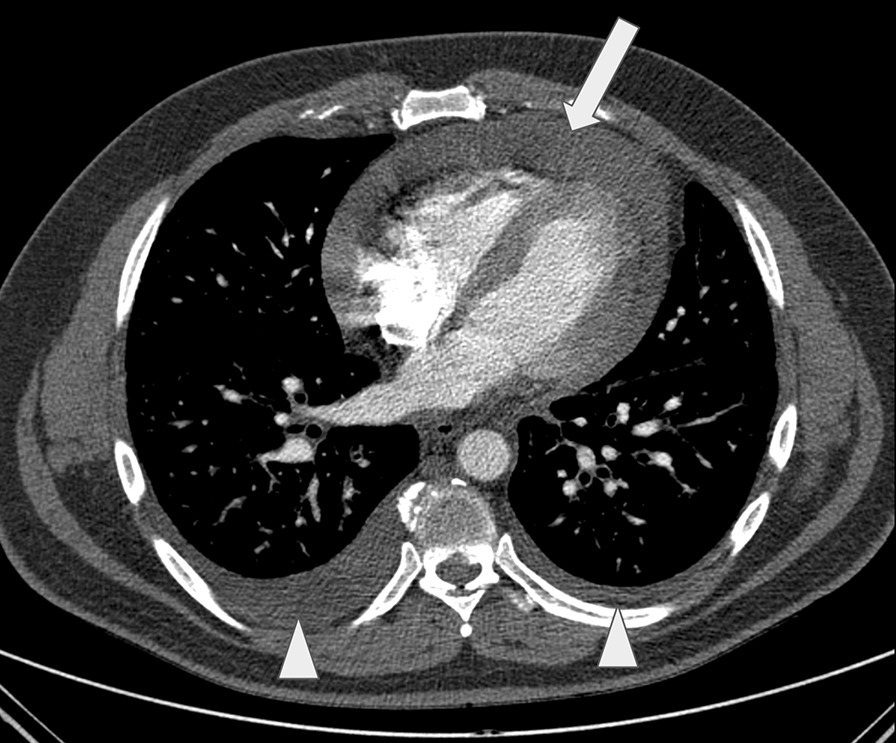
Contrast-enhanced chest computed tomography at the level of the inferior pulmonary vein shows moderate pericardial effusion (arrow) and small bilateral pleural fluid (arrowheads)
